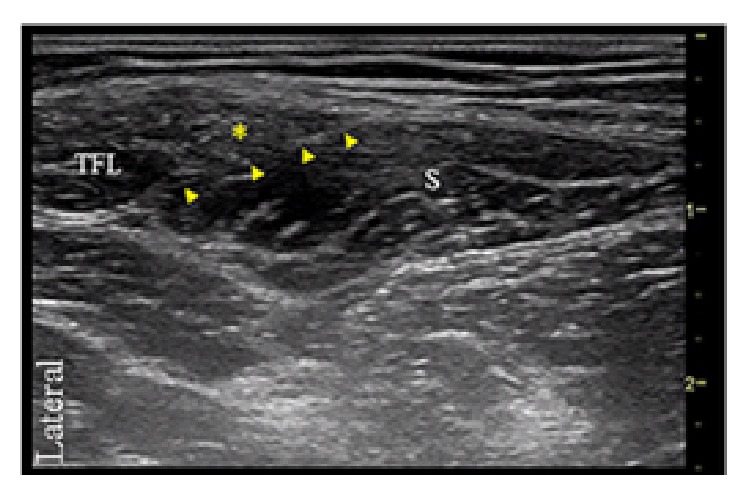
Transverse ultrasound image of the lateral femoral cutaneous nerve (asterisk) lying below fascia lata and lateral to the sartorius muscle (S) and medial to the tensor fasciae latae muscle (TFL). Arrow heads point to the fascia that separates the sartorius muscle from the LFCN.
